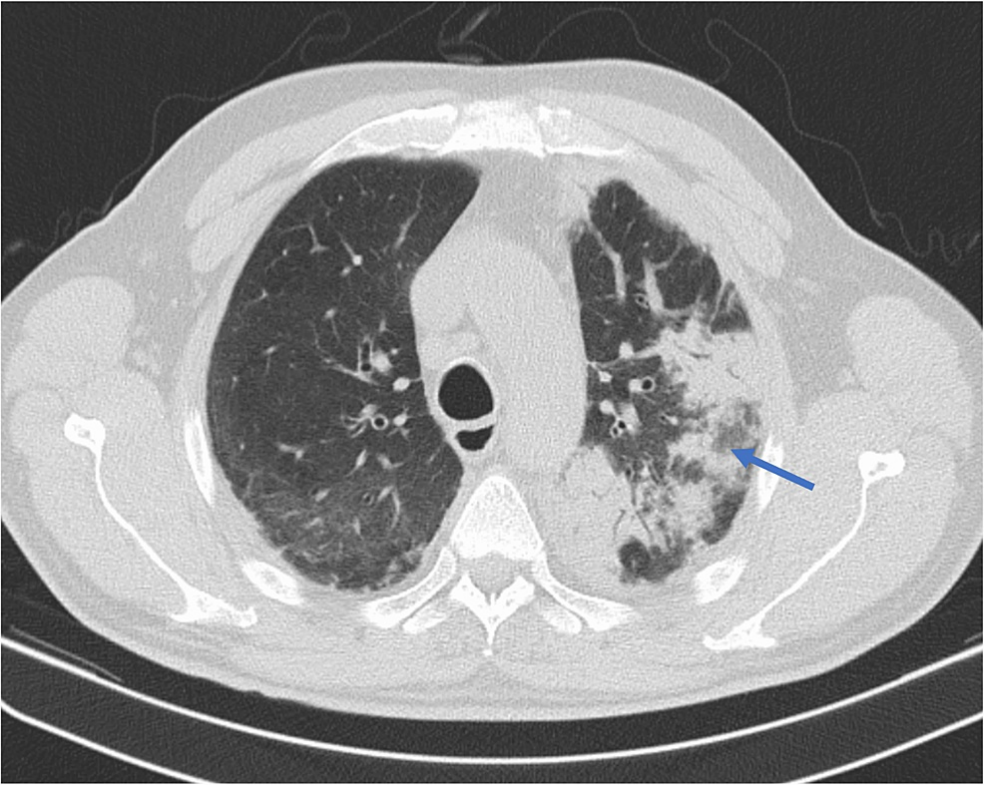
Admission CT showing consolidation of the lung parenchyma
